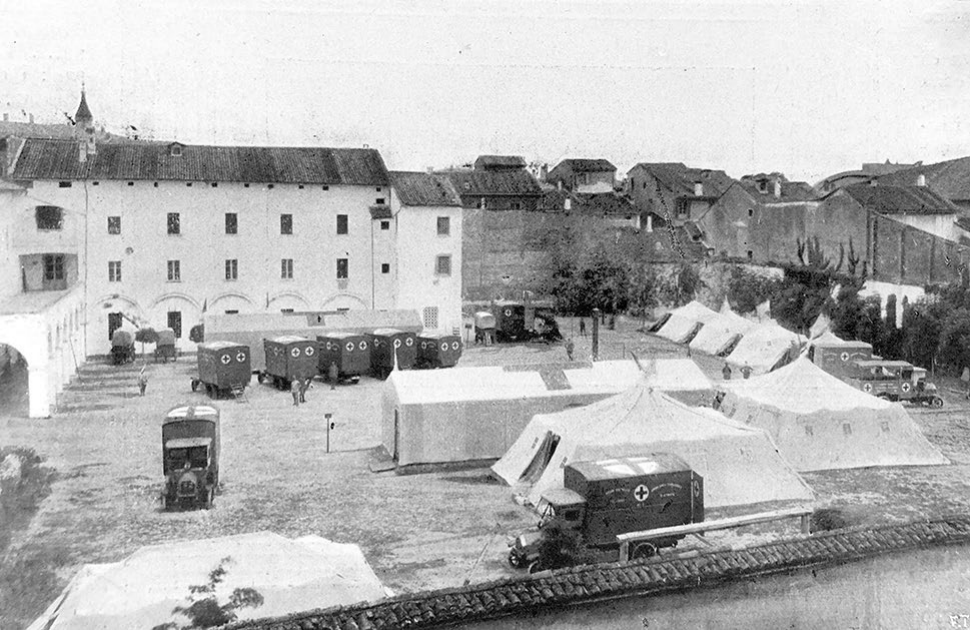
An Army Surgical Ambulance deployed in the courtyard of Caserma Masino in Bologna in May 1916 before departing for the frontline. The long rectangular tent hosted the theatre and X-rays apparatus
